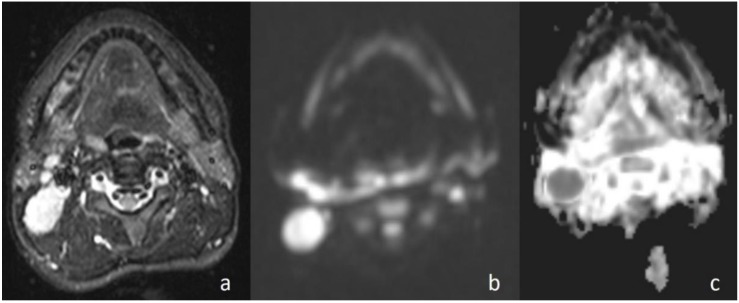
Non-Hodgkin lymphoma. (a) Axial T2WI shows metastatic cervical lymph node nearby SCM; (b) the lymph node, on the right side of the neck at level of lateral cervical region, shows high signal intensity on DWI; and (c) the mass is hypointense on the ADC map (ADC value of 0.62 × 10^−3^ mm^2^/s).
